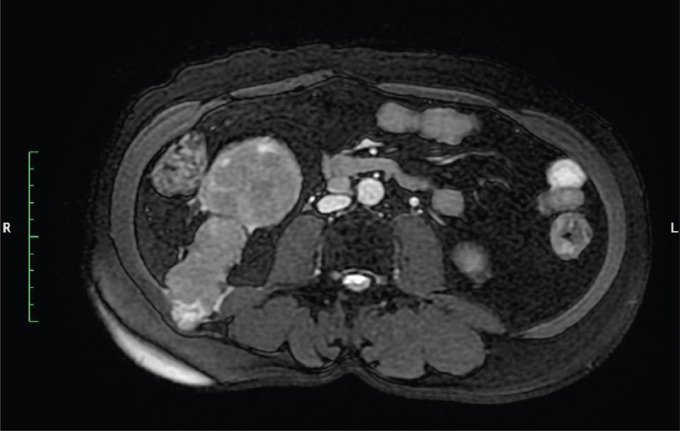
MRI abdomen axial T2 FATSAT image show tract of PCNL from lower pole kidney to abdominal muscles.
